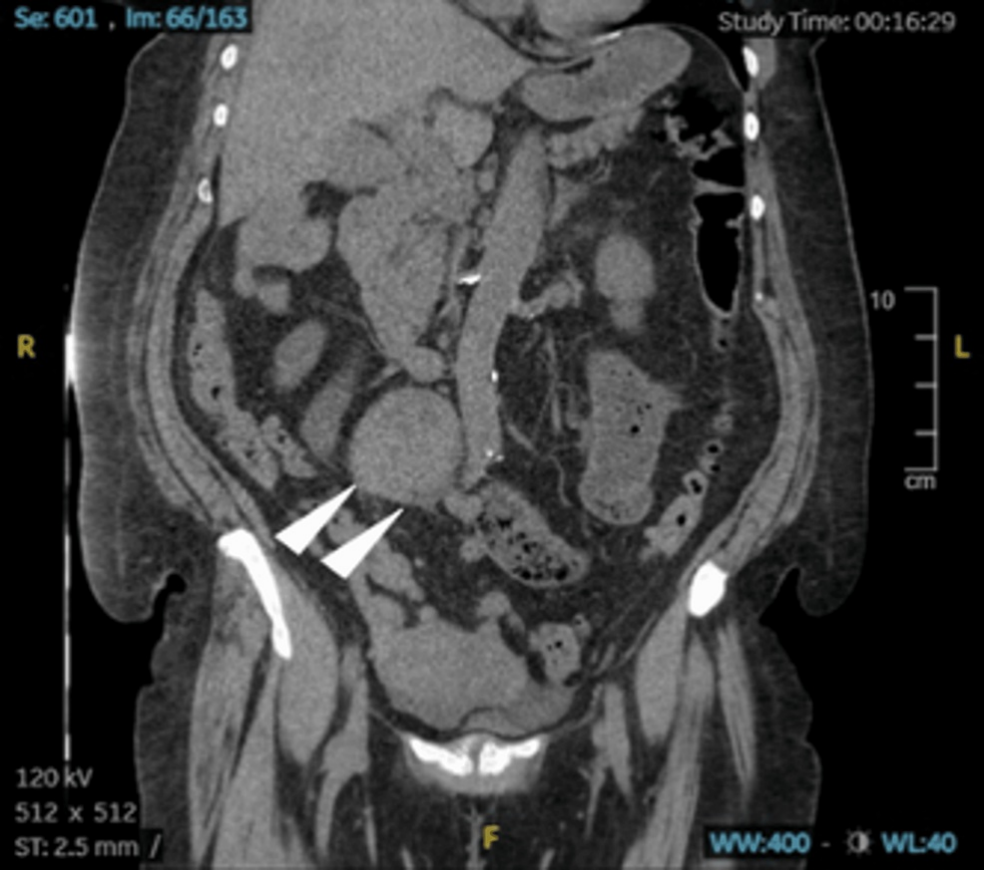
CT of the abdomen and pelvis without contrast, supine position, coronal view displaying distended loops of small bowel, and adhesions near the NET (arrowheads) taken at initial ER presentation. NET: neuroendocrine tumor
